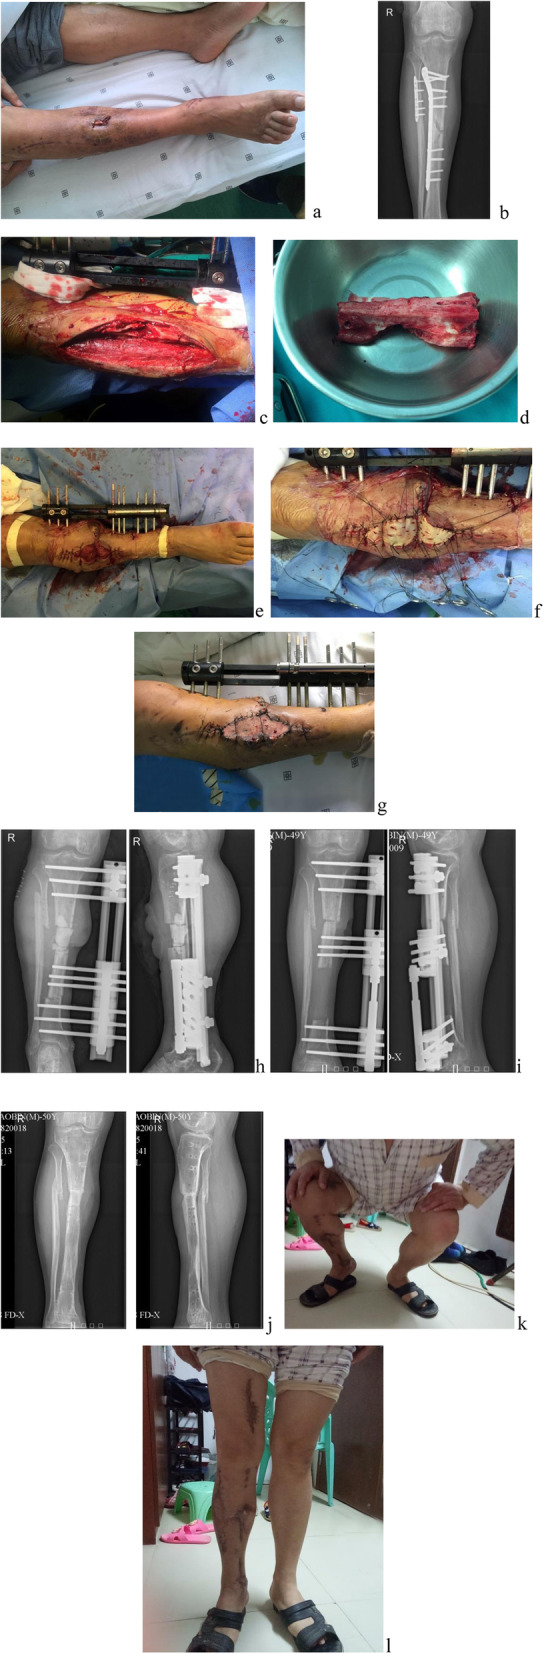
Case 2. A 49-year-old male patient with multiple open fractures of the right tibia and fibula (Gustilo grade II) due to a traffic accident underwent emergency debridement and internal fixation in a local hospital for half a year. Deep infection occurred after the operation, which was still uncontrollable after three times of debridement. Sinus tract was observed on the medial side of the lower extremity with pus (a). X-ray showed the tibia and fibula defect and sclerosis in part edge (b). Extended debridement, internal fixator removal, infected bone segment excision (approximately 9 cm), external fixation, tibiofibular shortening, distal tibial osteotomy, and full-thickness skin grafting were performed (c–f). At 1 week after operation, the wounds healed well, and the skin graft survived (g). Postoperative X-ray showed the tibial defects with good alignment (h). At 4 months after bone transport, the bone ends were contacted and the patient began weight-bearing walk (i). At 13 months after the operation, bone fracture healed well, and the external fixator was removed to restore normal walking function. (j–l). After 24 months of operation, the ASAMI functional score of the affected extremity was excellent, with an external fixation index 1.44
